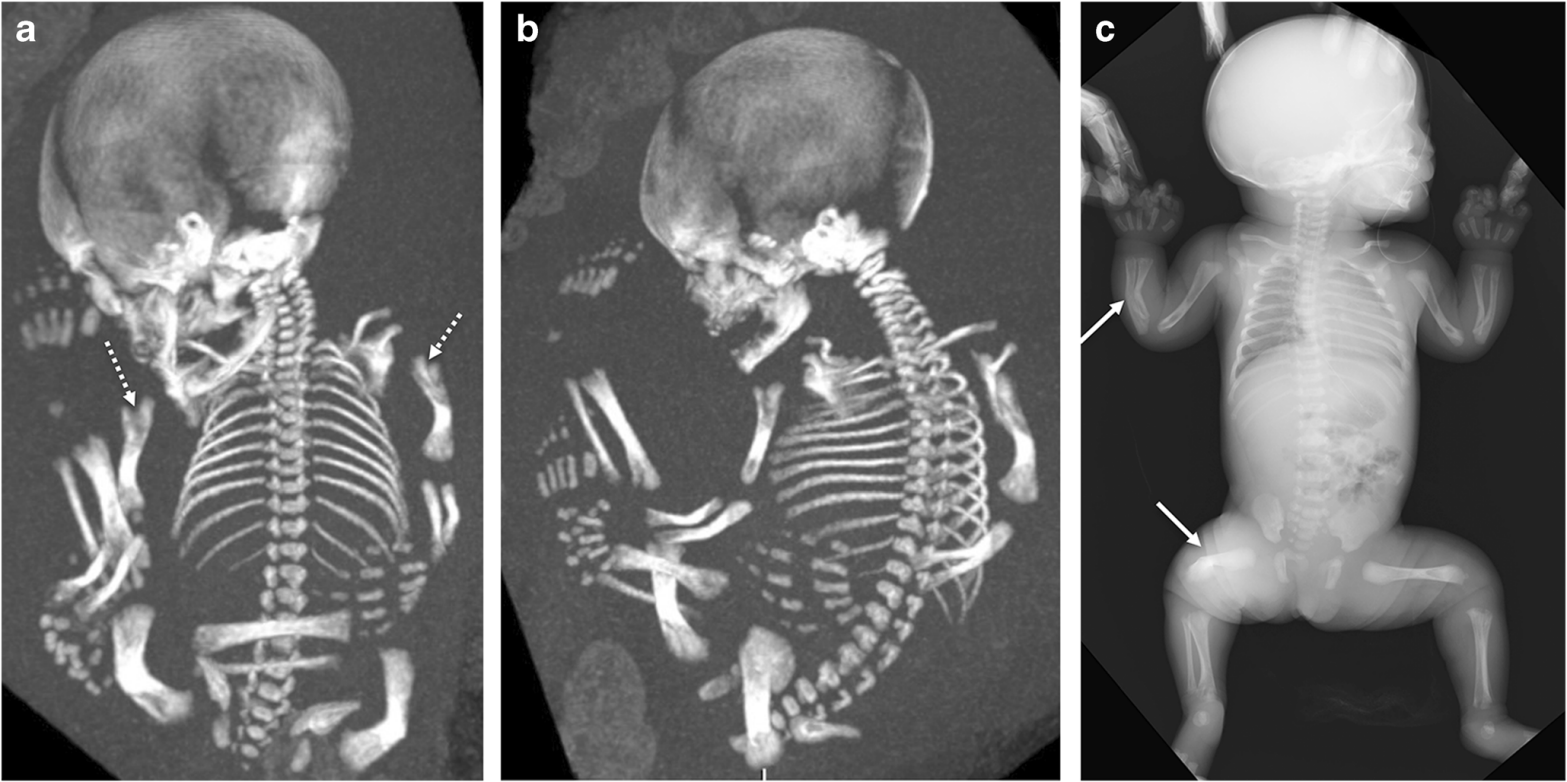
Images of a girl with a regressing phenotype of perinatal hypophosphatasia at 30 weeks’ gestation*.*a,b Three-dimensional fetal CT scans in the coronal view (a) and sagittal view (b) show metaphyseal “tongues” of radiolucency (*dashed arrows*) and bowing of the long bones. c An anteroposterior radiograph obtained at birth shows regression of the metaphyseal change, although bowing persists (*arrows*)
